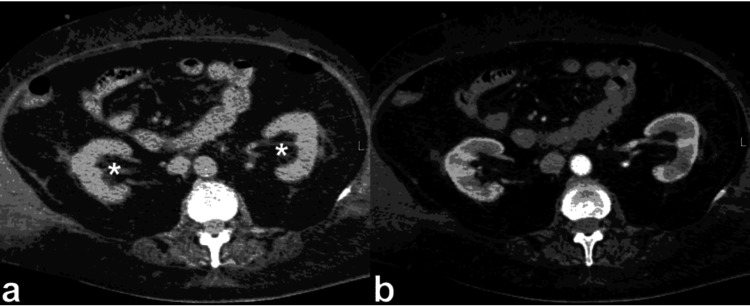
Non-enhanced CT (NECT) phase (a) and the contrast-enhanced CT (CECT) arterial phase (b) showcase the presence of extra pure fat replacement surrounding both sides of the renal sinus, depicted by the asterisk. However, despite the presence of this fat replacement, there is no discernible mass effect and no displacement observed on the urinary collecting system. This figure is a republished image, permission was obtained from the original publisher, https://epos.myesr.org/poster/esr/ecr2018/C-2802
